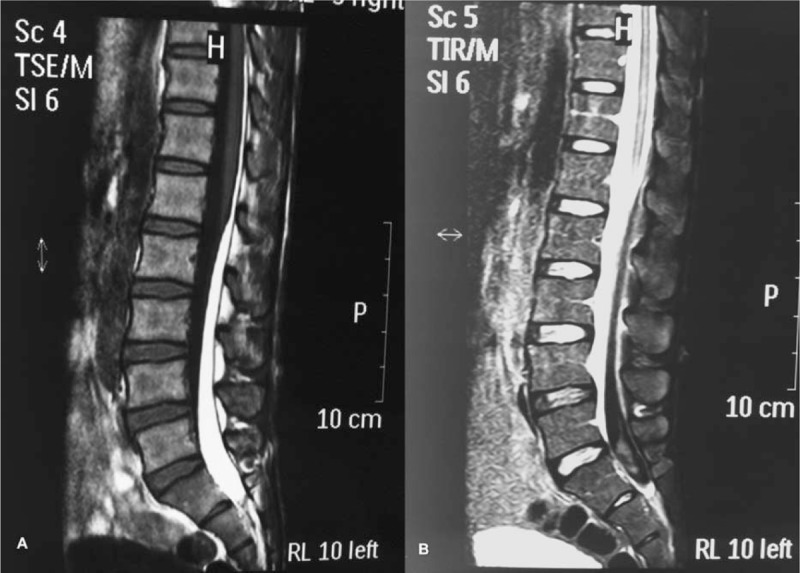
MRI scan of the lumbar spine at local hospital. Sagittal T1-weighted (A) and T2-weighted (B) images revealed a large subdural hematoma, extending from L1 to S1, presents as hyperintense signal on T1 weighted sequences and hypointense to isointense signal on T2 weighted sequences. MRI = magnetic resonance imaging.
